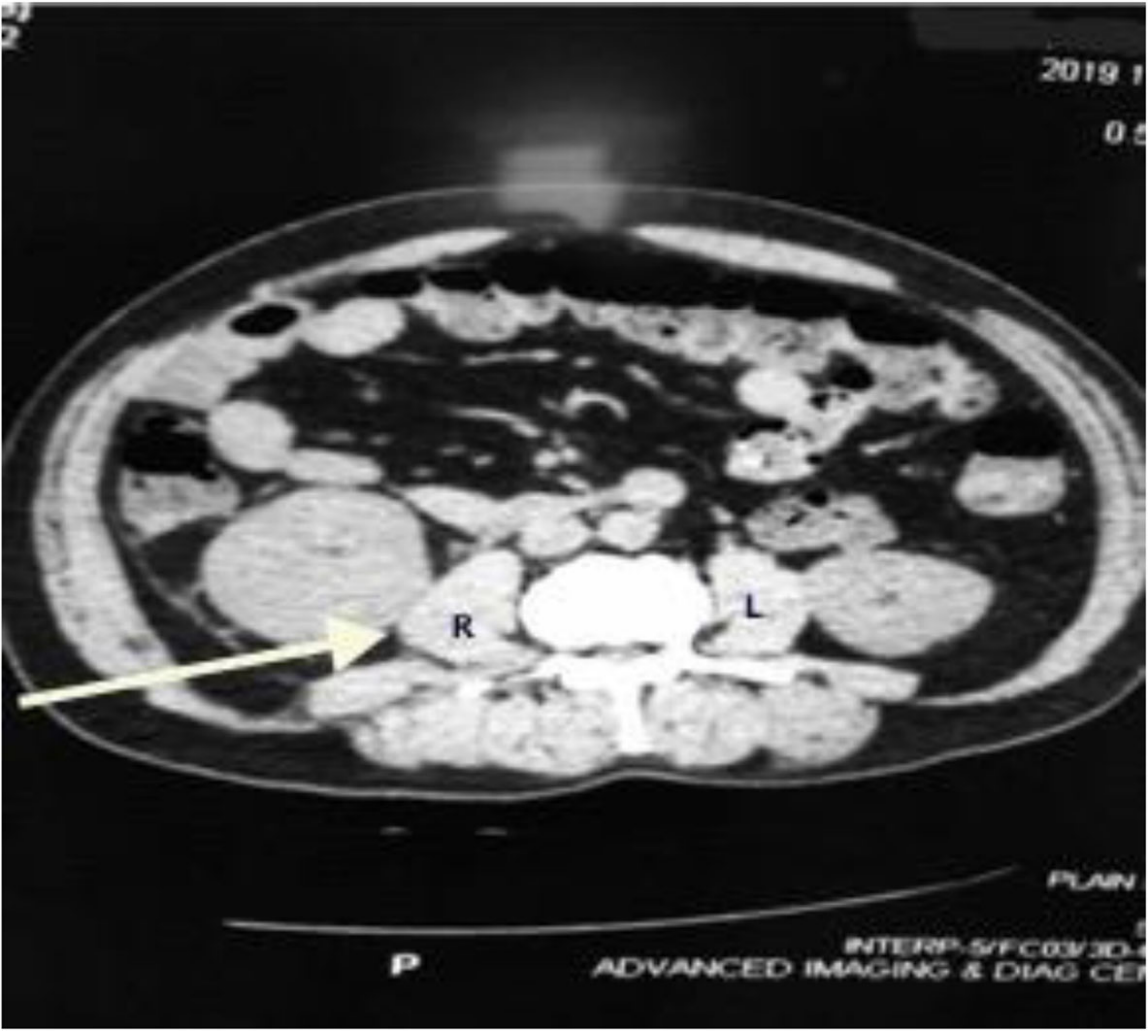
CT scan kidneys-ureters-bladder findings (on Day 5 of illness). Right kidney (R) has perirenal haziness and fatty stranding (arrow); thickened right lateral conal fascia with minimal surrounding haziness; tiny renal concretions, spenunculus, and plate atelectasis in the postero-basal segment of right lower lobe; mild degenerative changes visualized in spine. Left kidney (L) is normal
